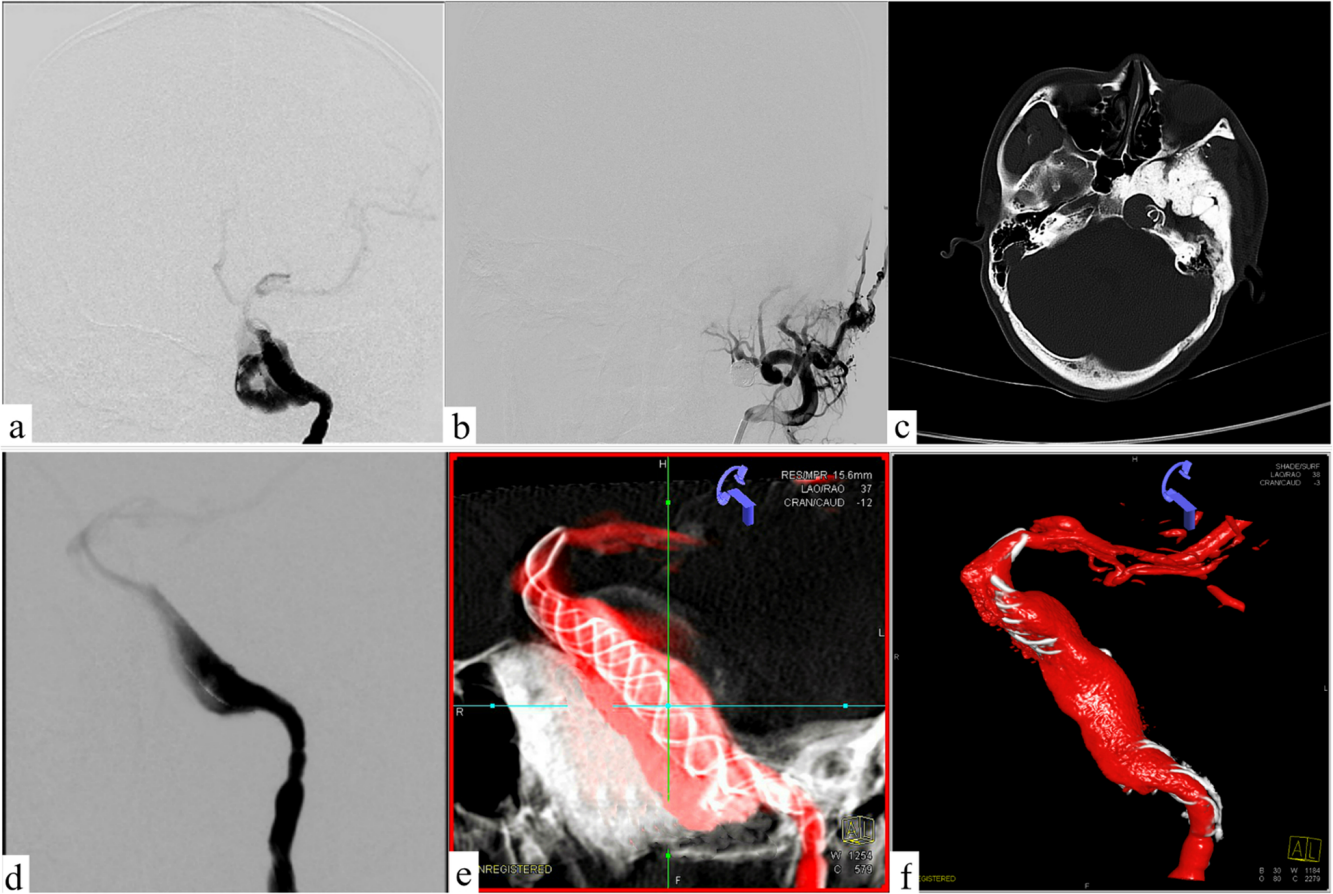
Postoperative follow-up of CT and DSA. a Left internal carotid artery angiography shows the redirection of the aneurysm inflow jet. b External carotid arteriography shows complete occlusion of MA. c Postoperative reexamination of CT. d DSA reveals the variation of flow direction in the stents. e–f 3D-DSA display the transform volume and morphology of GSA
